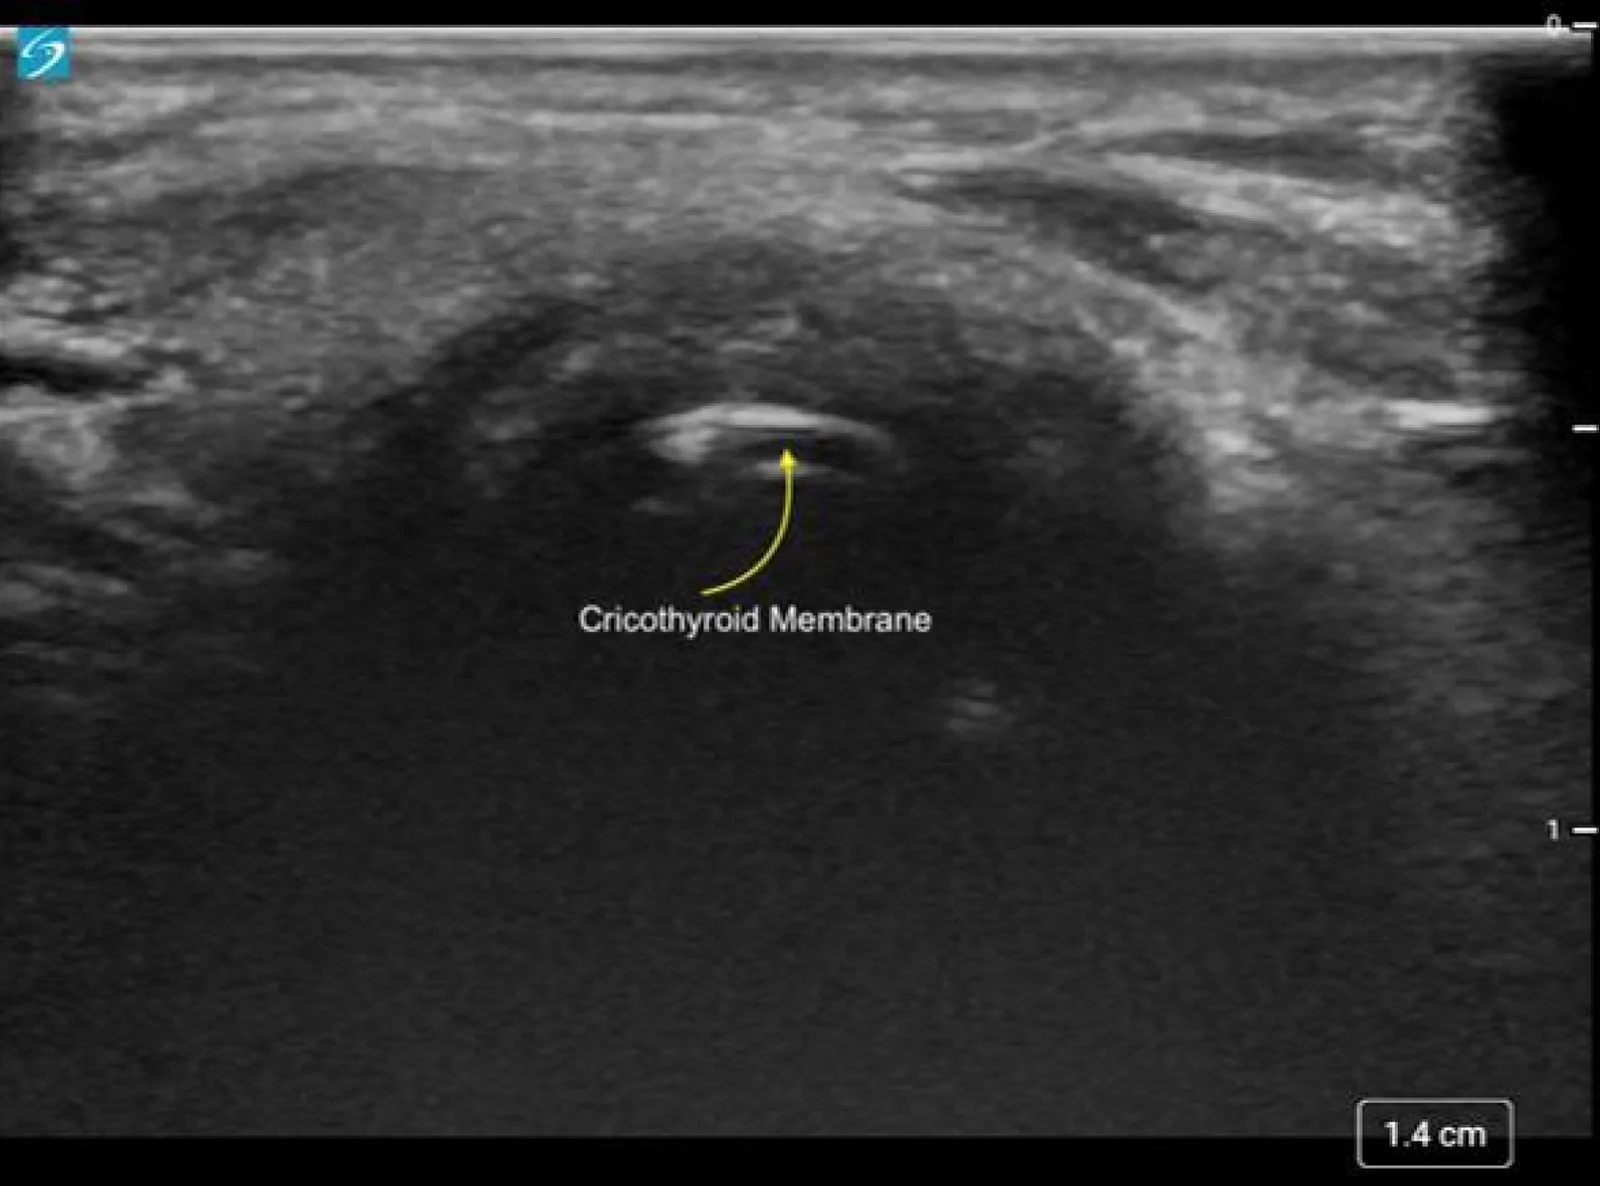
Transverse ultrasound of the neck demonstrating the cricothyroid membrane.
